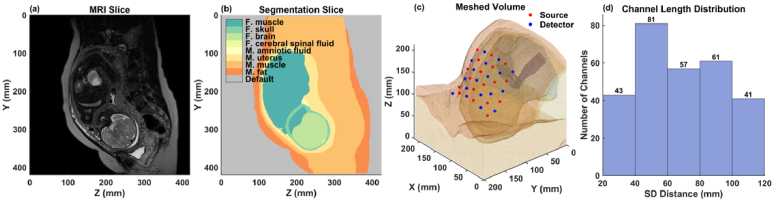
Fetus in Occiput Anterior (OA) position. (a) Sagittal MRI slice. (b) Segmentation of (a). (c) 3D mesh with SD grid on the maternal abdomen. (d) Channel length distribution of the SD grid in (c).
